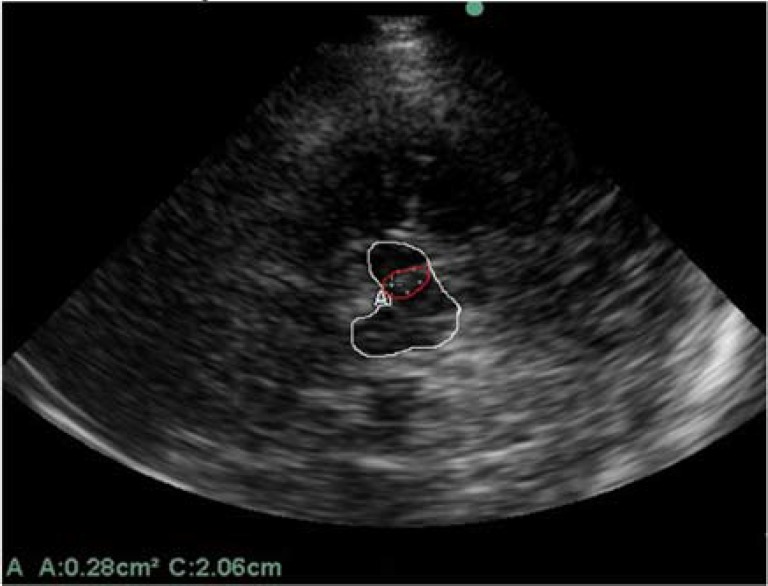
Transcranial sonography (TCS) of midbrain and maximal area of substantia nigra (SN) hyperechogenicity
